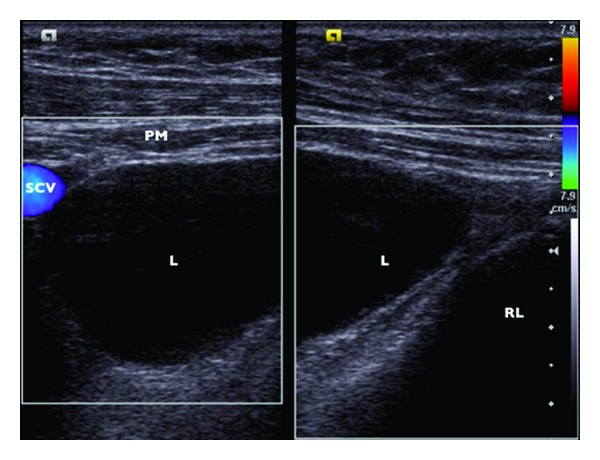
Color doppler ultrasound, axial view. PM: pectoral muscle; L: lymphocele; RL: right lung; SCV: subclavian vessels.
